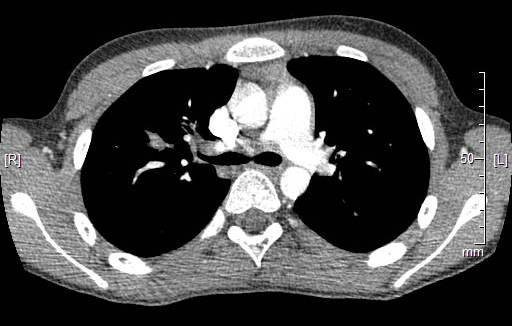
Chest CT confirms the neoplasm in the right lung.
